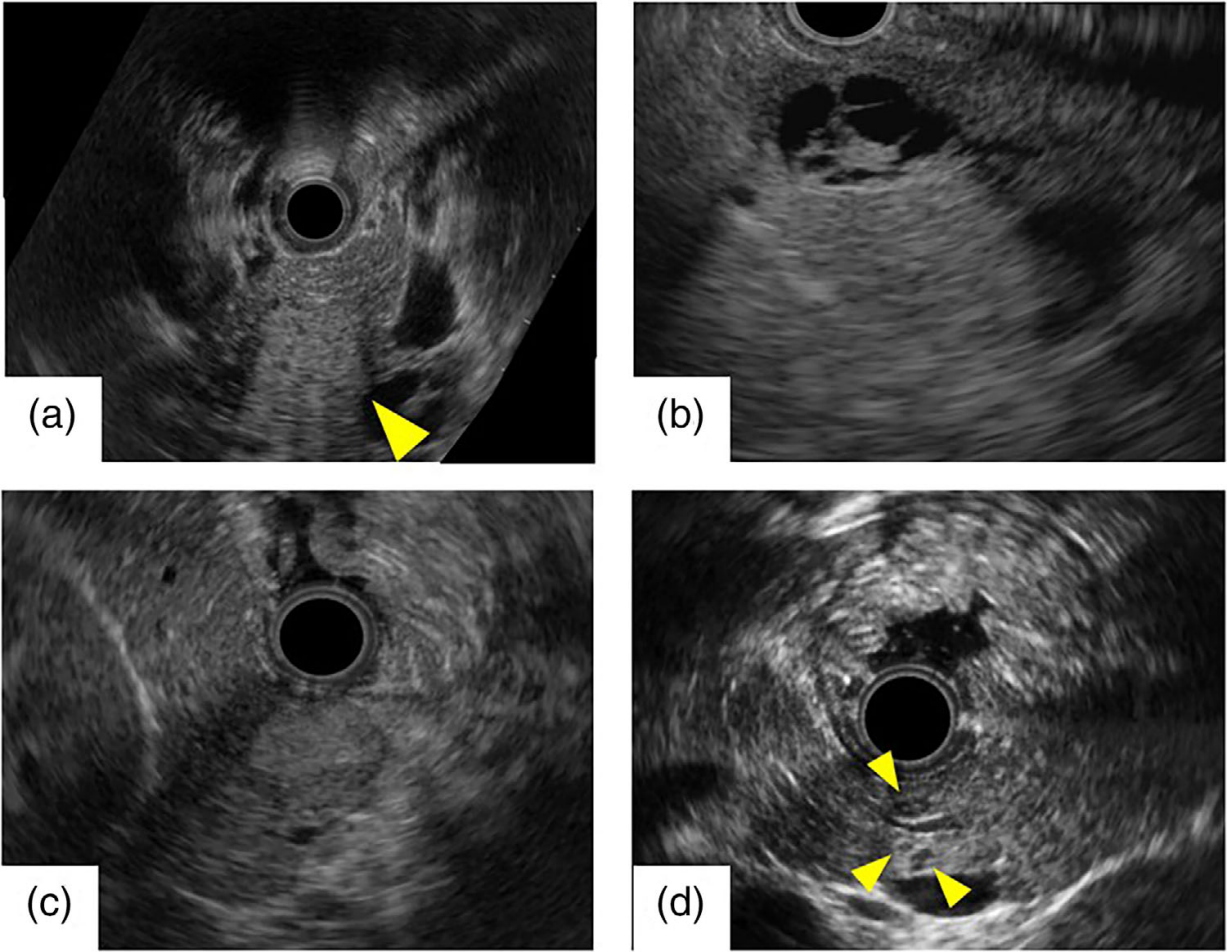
Endoscopic ultrasound. (a) A poorly demarcated hyperechoic lesion that appeared to contain a collection of small cysts was evident in the pancreatic head (yellow arrowhead). (b) A multilocular cystic lesion with thick septa was evident in the pancreatic body. (c) A well‐demarcated, hyperechoic mass was evident in the pancreatic tail. Its interior was homogenous, and it was considered to be a solid mass. (d) Well‐demarcated, isoechoic to hyperechoic lesions a few millimeters in size that had not been identified on contrast‐enhanced computed tomography were present in the pancreas (yellow arrowhead).
